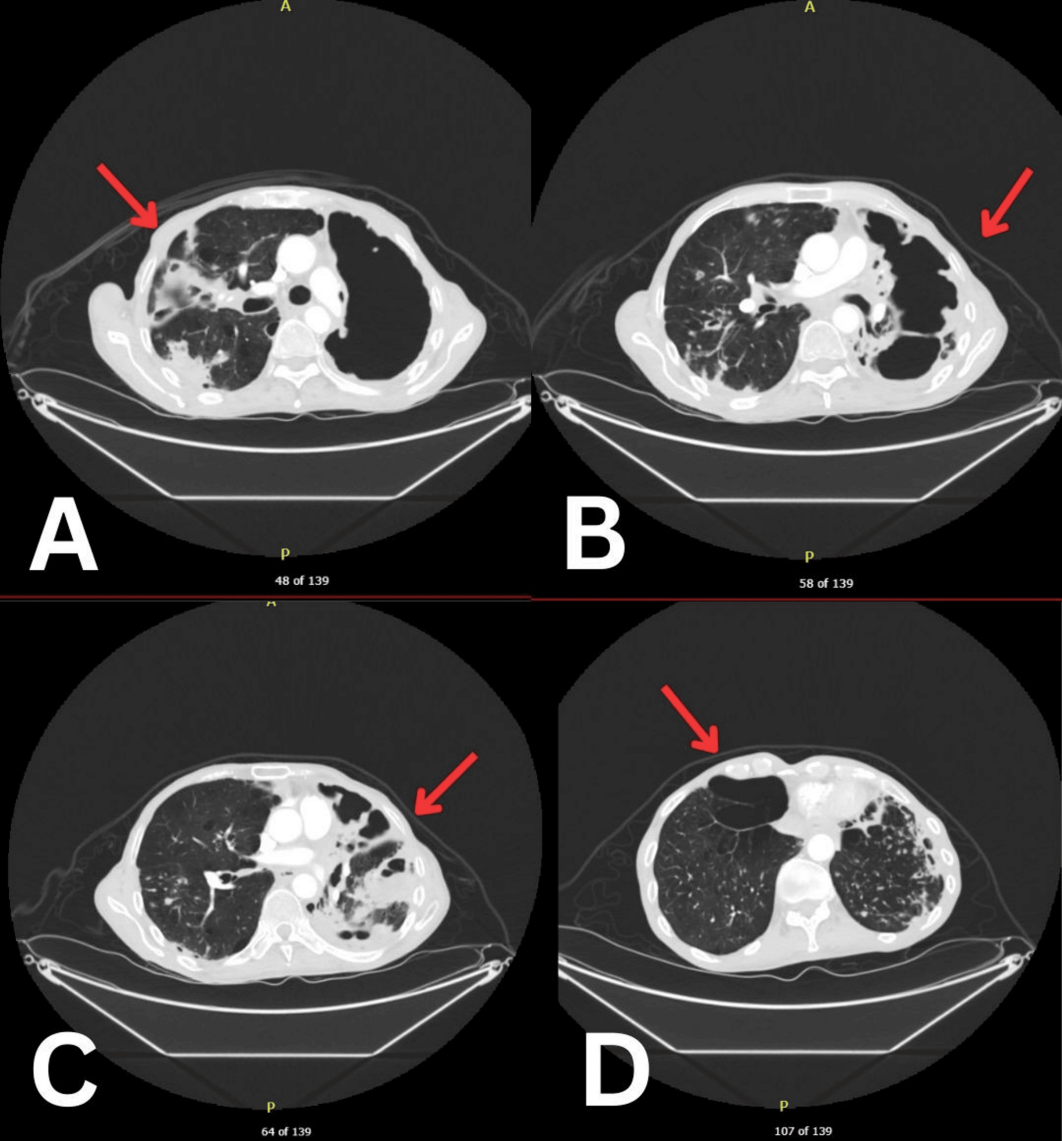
CT-Thorax with views at different levels from cephalic (A) to caudal (D) with left and right-sided cavitating disease (arrows).
